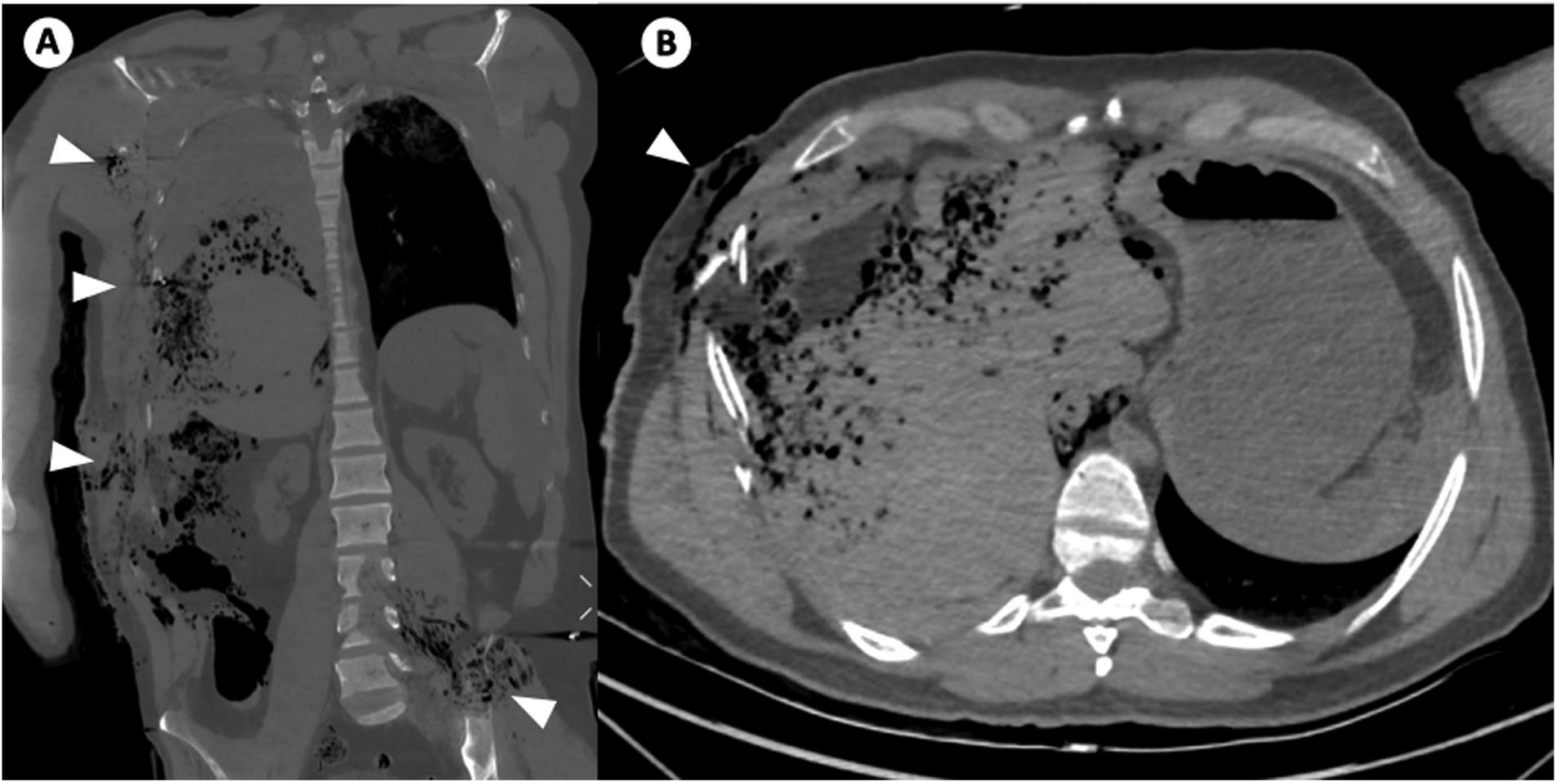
Coronal postmortem computed tomography (PMCT) image of the chest and abdomen (A) and axial PMCT image at the level of the upper abdomen (B) of a victim with multiple ballistic impact sites (arrowheads). Partially overlapping and dispersing trajectories complicate the identification of different ballistic trajectories.
